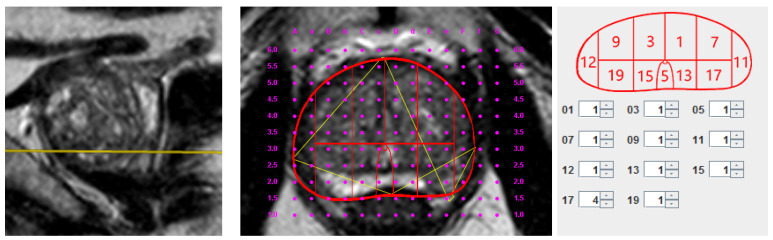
Multiparametric magnetic resonance imaging (mpMRI) image adjusted to brachytherapy grid coordinates with a positive Prostate Imaging Reporting and Data System (PI-RADS) 4 lesion. PI-RADS v2 score of 4 in zone 17 of the modified Barzell scheme, corresponding to coordinate E2.0 in the brachytherapy grid (urethra in coordinate D2.5).
